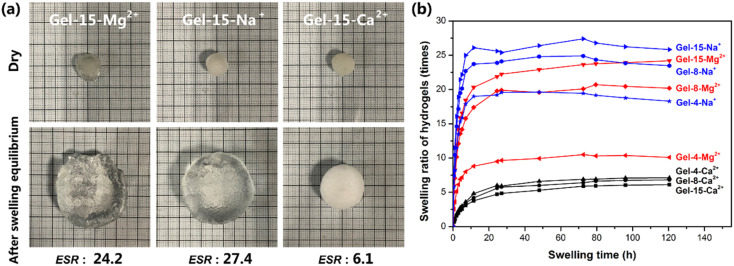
(a) Appearance of MCl_*n*_-doped PDMA-*g*-PSMA hydrogel before and after swelling. (b) Swelling curves of MCl_*n*_-doped PDMA-*g*-PSMA hydrogels.
